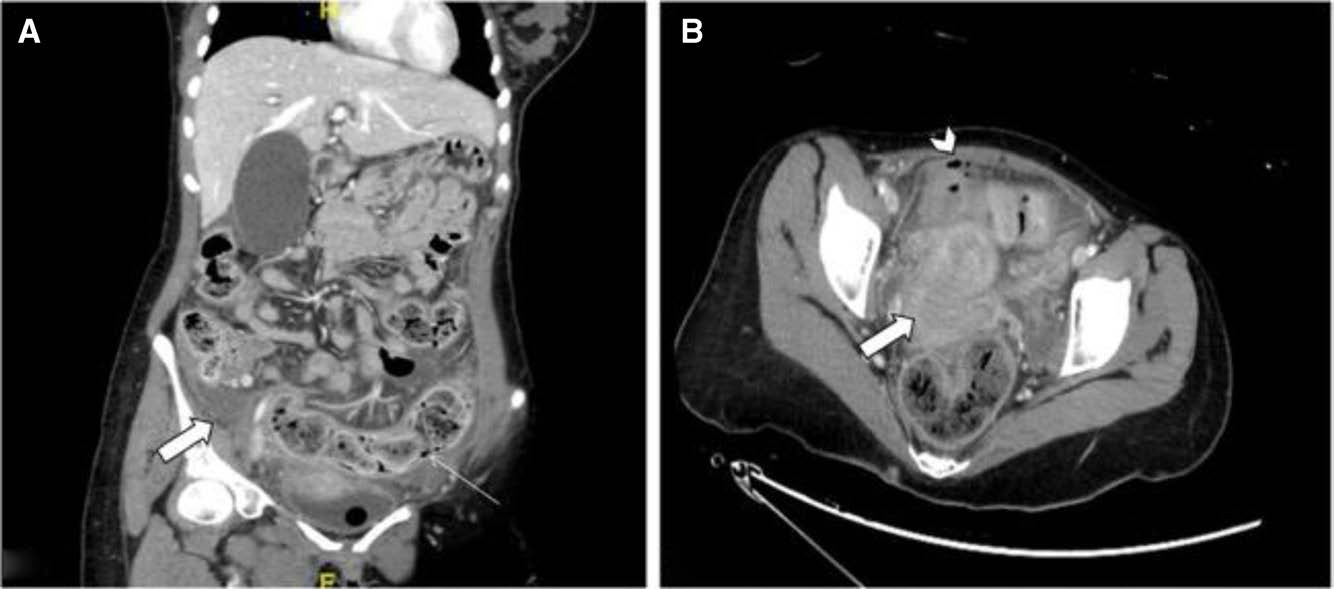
CT abdomen images: (A) coronal view with findings of sigmoid colon loaded with stool (small arrow) and a small amount of abdominopelvic fluid (large arrow). (B) axial view with results of pelvic fluid (large arrow) and foci of pneumoperitoneum (arrowhead).
